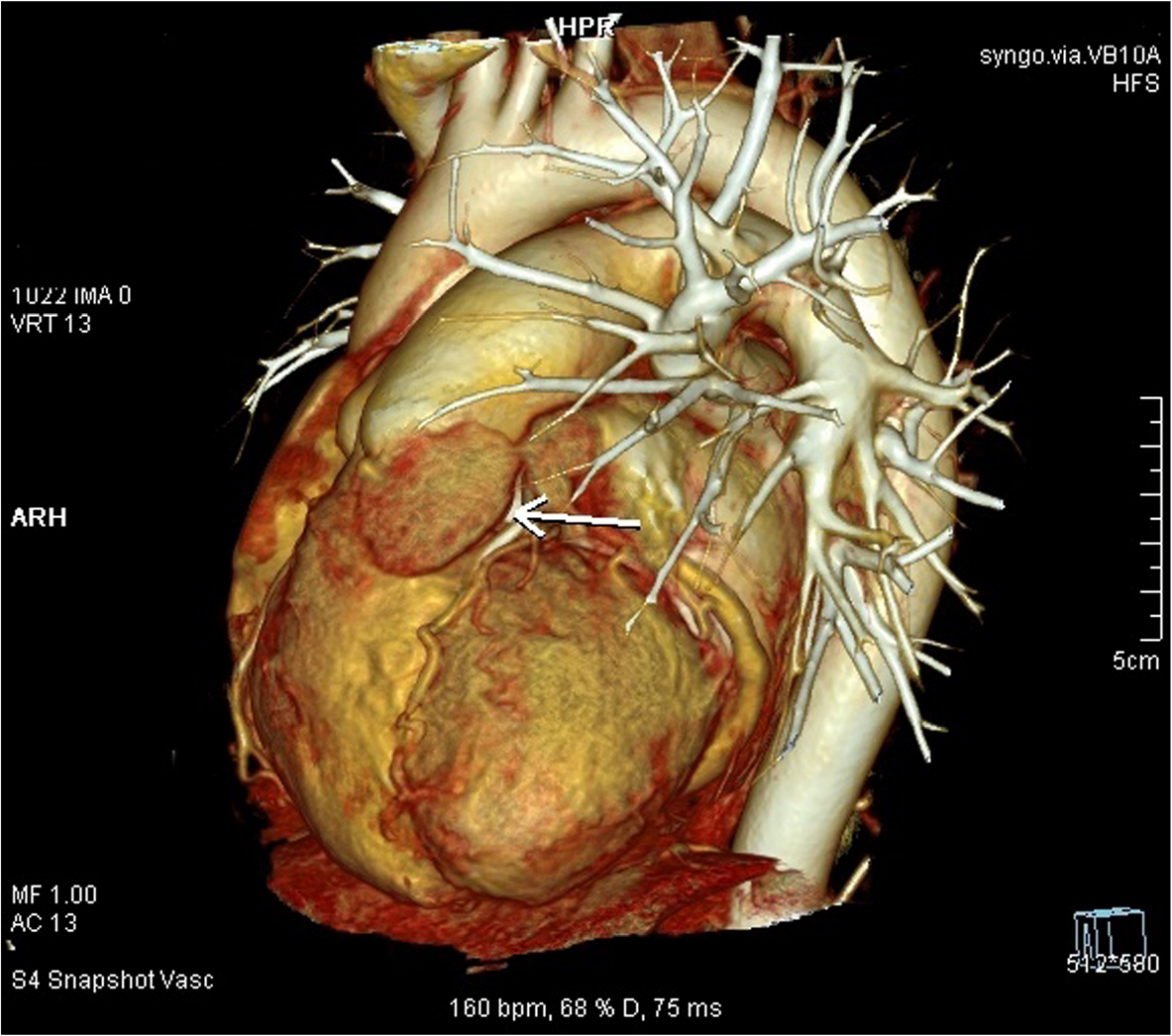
Cardiac 3D CTA reconstruction. The white arrow points to the mass
